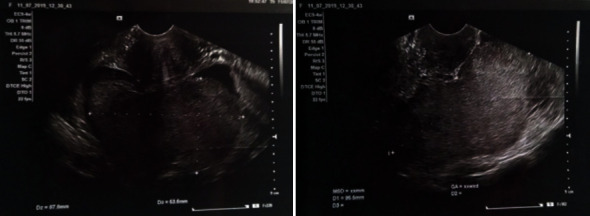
Abdominal Ultrasonography (US) in the emergency room revealed an echogenic mass on the pouch of Douglas.
